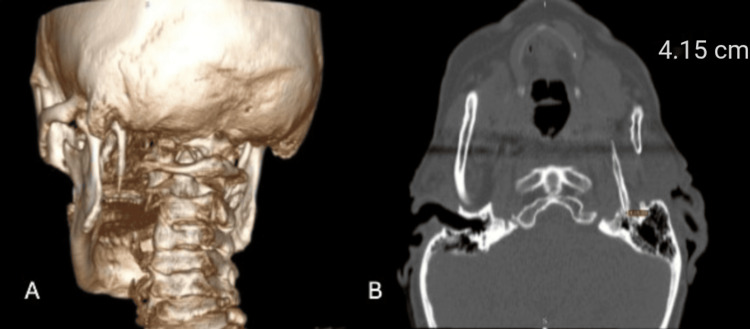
Volumetric craniofacial CT reconstruction showing the left styloid process (A). Measurement of the length of the styloid process (B).
